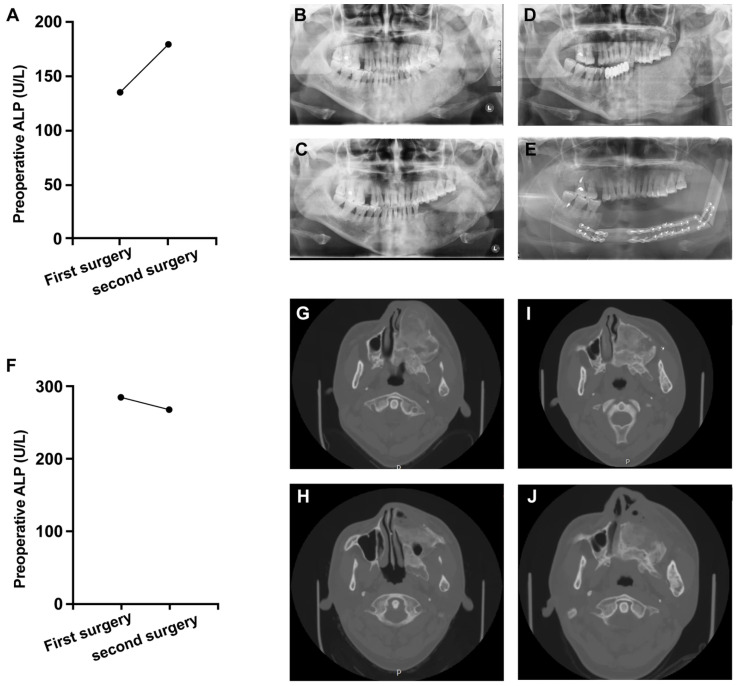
Two cases of patients with active lesion progression. (A) Preoperative ALP levels at the time of the first and second surgeries in a female patient with fibrous dysplasia in the bilateral mandible. (B) Preoperative panoramic radiograph from the first surgery showing a ground-glass, ill-defined mass in the mandible (Date: 12 August 2014). (C) Postoperative radiograph following the first surgery (Date: 29 August 2014). (D) Preoperative radiograph from the second surgery showing significant progression of the lesion compared to image (C) (Date: 4 September 2019). (E) Postoperative radiograph following the second surgery (Date: 16 September 2019). (F) Preoperative ALP levels at the time of the first and second surgeries in a male patient with unilateral polyostotic fibrous dysplasia in the left maxilla, sphenoid bone, ethmoid bone and left mandible. (G) Preoperative panoramic radiograph from the first surgery showing a ground-glass, ill-defined mass in the left craniofacial regions (Date: 12 March 2015). (H) Postoperative radiograph following the first surgery (Date: 17 April 2015). (I) Preoperative radiograph from the second surgery showing significant regrowth of the lesion compared to image (H) (Date: 18 May 2018). (J) Postoperative radiograph following the second surgery (Date: 25 May 2018).
